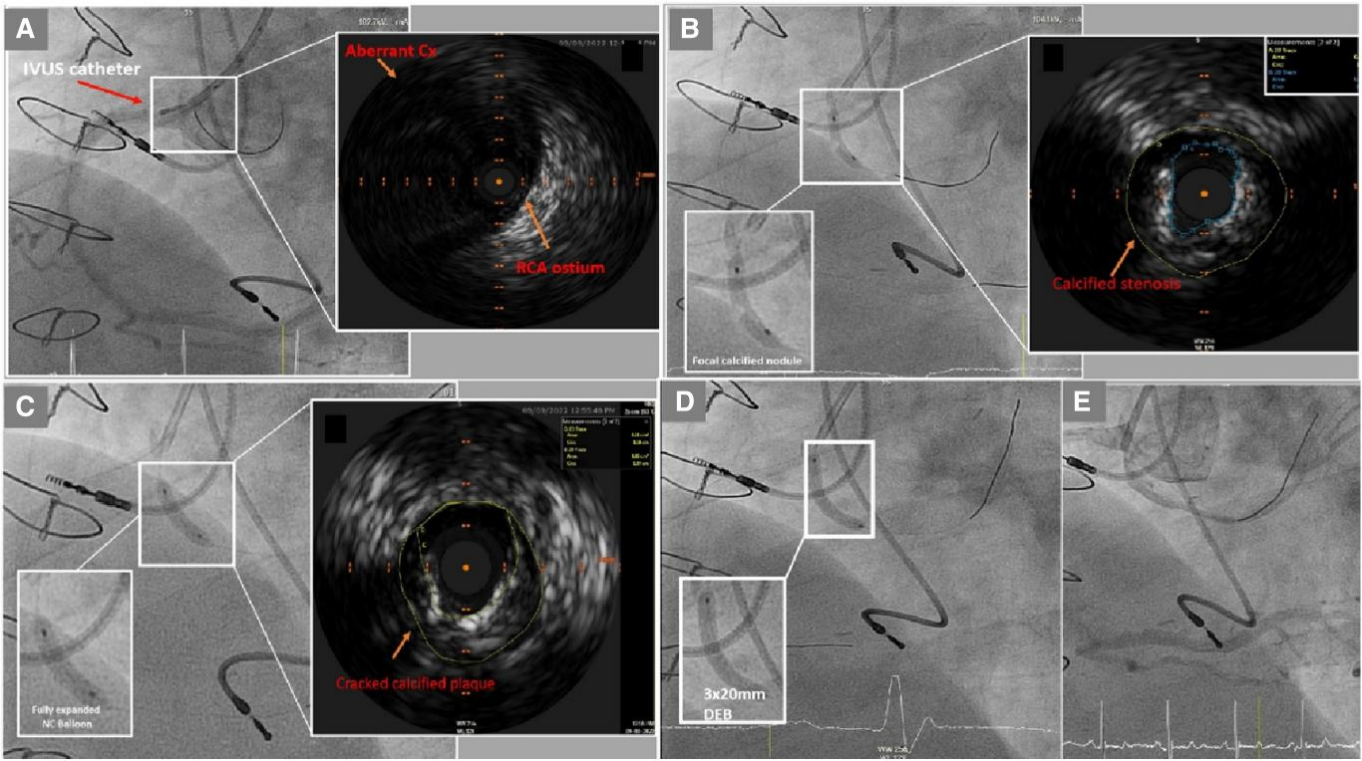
(*A*) Left anterior oblique (LAO) cine projection showing an intravascular ultrasound (IVUS) catheter placed at the RCA ostium in the right coronary sinus of Valsalva. The IVUS reveals the ostium of the anomalous LCX artery positioned at the 11 o’clock position relative to the RCA ostium. (*B*) LAO cine projection demonstrating an under-expanded 2.5 × 12 mm semi-compliant balloon with a focal calcified nodular lesion in the proximal anomalous LCX. The corresponding IVUS indicates severe calcified stenosis in the proximal anomalous LCX, with a minimum luminal area (MLA) of 3 mm² and a vessel area of 11 mm², reflecting a 73% reduction in luminal area. (*C*) LAO cine projection depicting a fully expanded 3.0 × 12 mm non-compliant balloon following the application of a 2.5 × 10 mm Coronary Cutting Balloon (Wolverine™, Boston Scientific). The IVUS shows an increased MLA of 6 mm² with cracked calcified plaque in the treated segment. (*D*) LAO cine projection demonstrating a well-expanded 3.0 mm × 20 mm drug-eluting balloon (SeQuent® Please NEO, B. Braun Melsungen AG, Germany) at 8 atmospheres (atm) within the proximal anomalous LCX. (*E*) Coronary angiogram in LAO projection showing a good final angiographic result following the application of the drug-eluting balloon therapy without any local complications.
